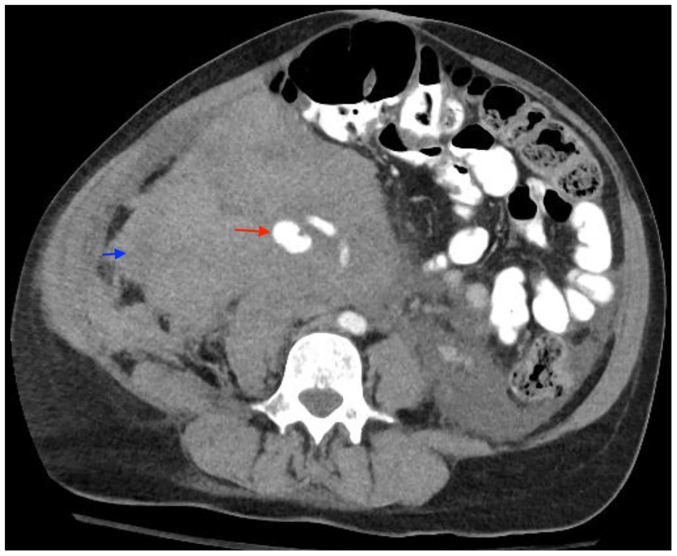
Computed tomography (CT) scan demonstrating right ovarian artery aneurysm (OAA) (*red arrow*) and associated retroperitoneal hematoma (*blue arrow*).
